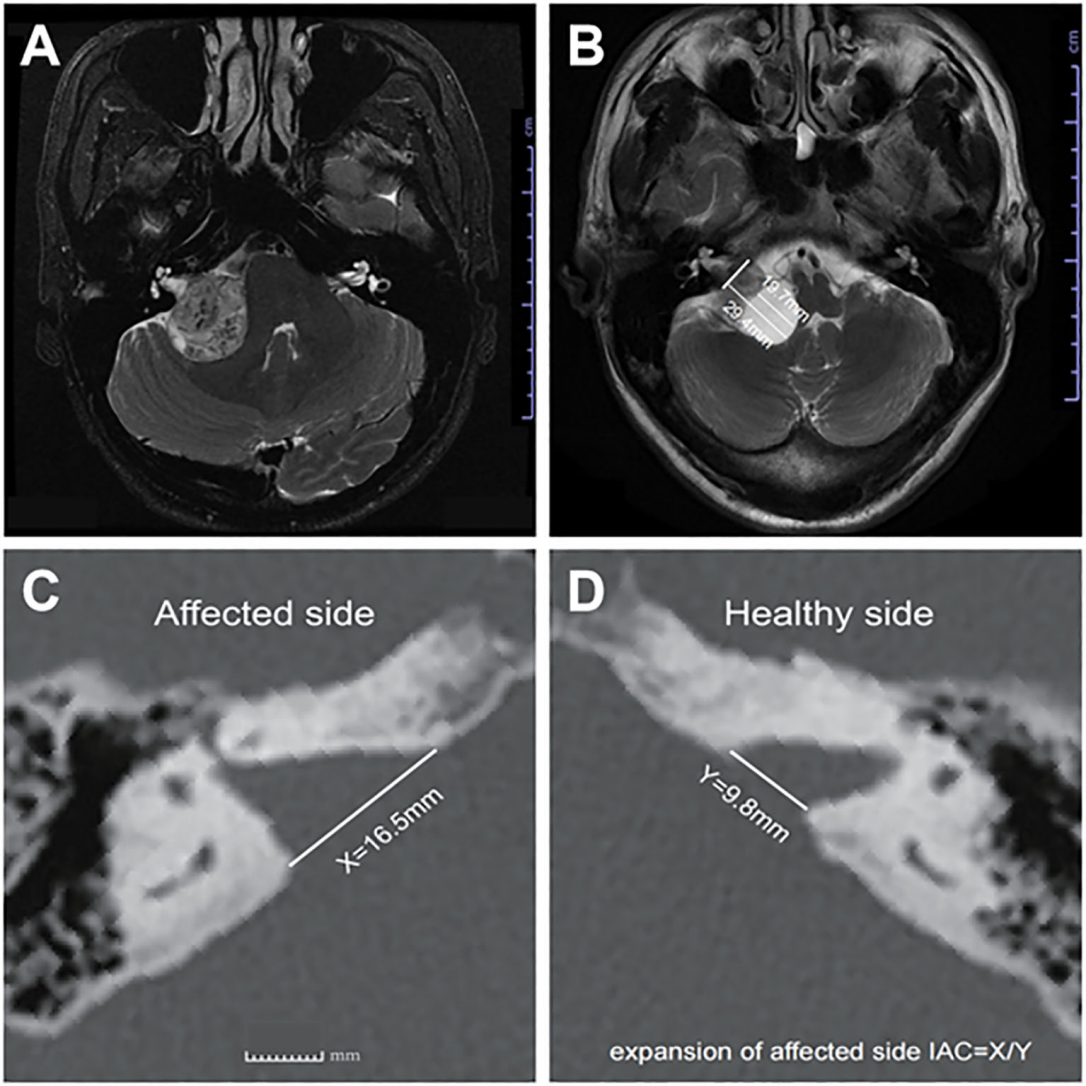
(A) Axial T2-weighted MRI image showing representative images of SVs, and (B) representative images of CVs, (C) shows the distance X from the affected side IAC nasal crest to the dorsal crest, and (D) the distance Y from the healthy side IAC nasal crest to the dorsal crest; X/Y = expansion of affected side IAC.
